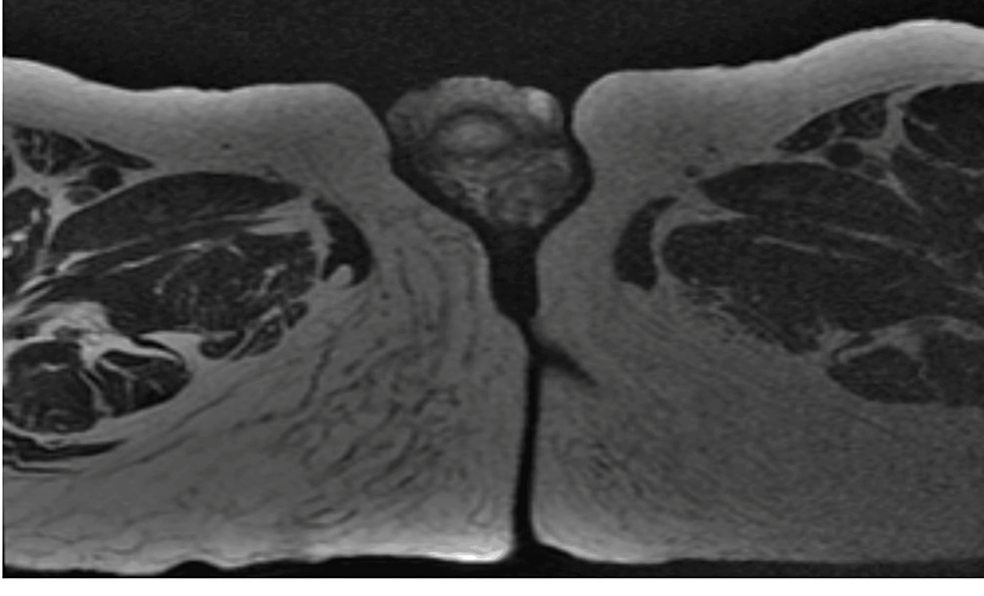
Axial pelvic MRI T2 showing tumor thickening centered on the glans of the penis, infiltrating the fascia and the spongy urethra
